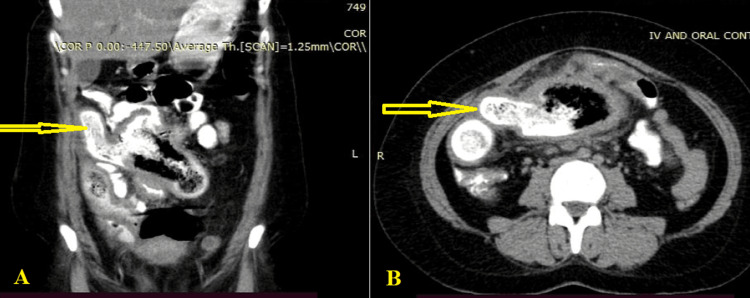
Computed tomography scan reveals a spongiform mass containing air bubbles measuring 6 × 9 × 5 cm in the intraperitoneal supraumbilical region, surrounded by a bowel loop with moderate proximal bowel dilatation suggestive of gossypiboma with partial intestinal obstruction (arrow); A: sagittal view, B: coronal view.
